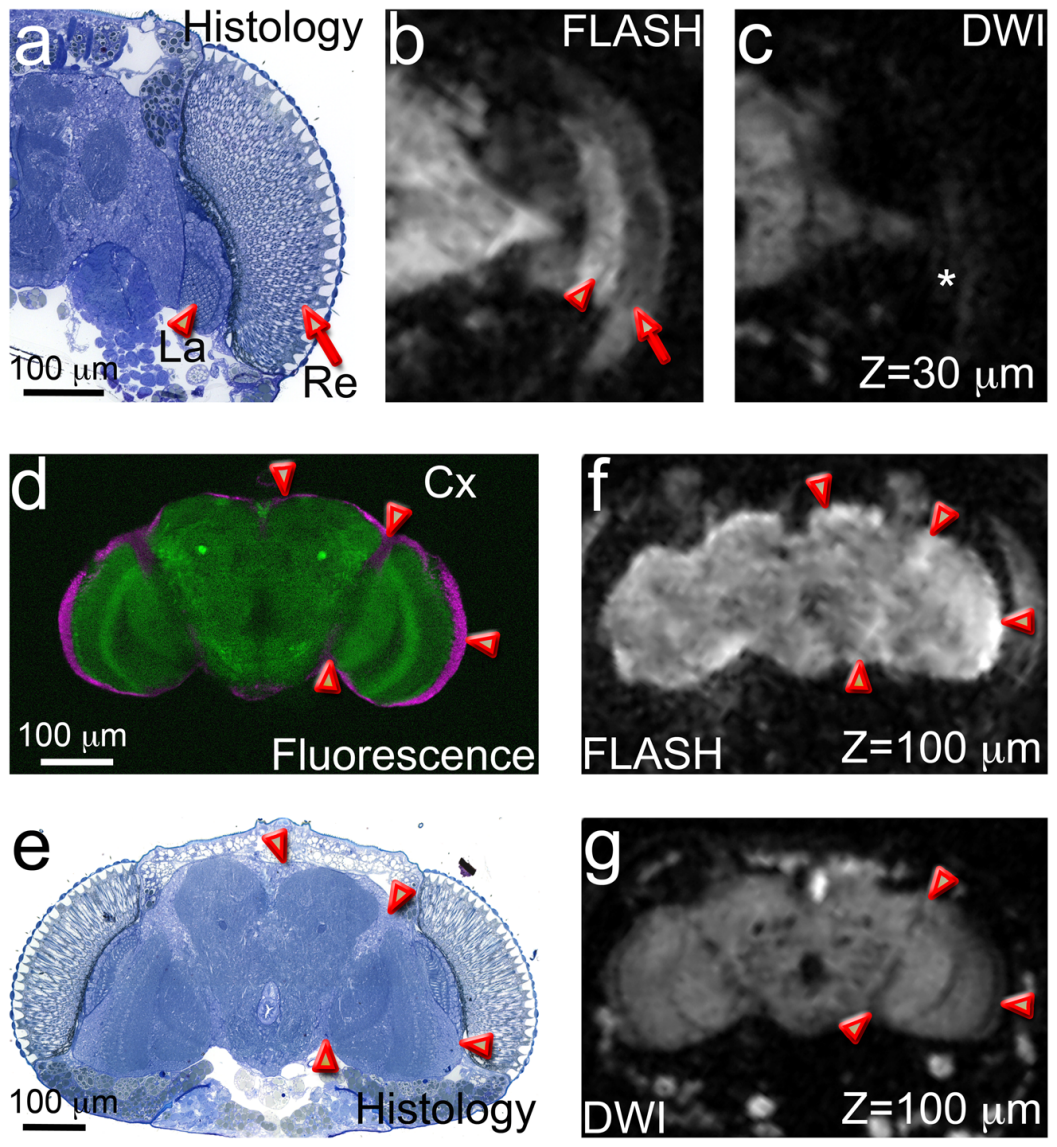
Example of FLASH and DWI microimages discriminating different head
